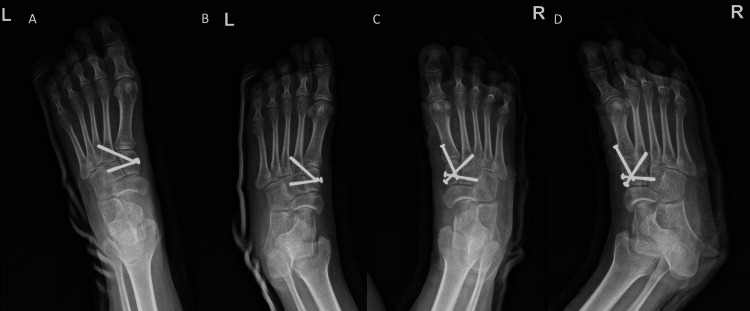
Immediate postoperative anteroposterior and oblique x-ray images of both feet (A) Left foot AP x-ray. (B) Left foot oblique x-ray. (C) Right foot AP x-ray. (D) Right foot oblique x-ray. AP: Anteroposterior.
